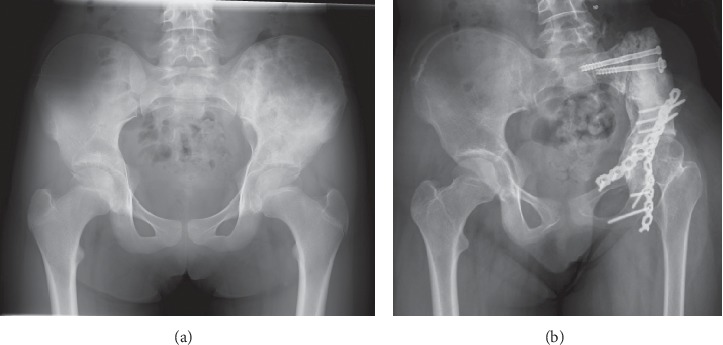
Case #19: 11-year-old male with Ewing sarcoma of the left pelvis. (a) Preoperative X-ray. (b) 6 years after radiograph of treatment with osteoarticular extracorporeal irradiated autograft. Osteonecrosis of the left femoral head occurred with acceptable functional results.
